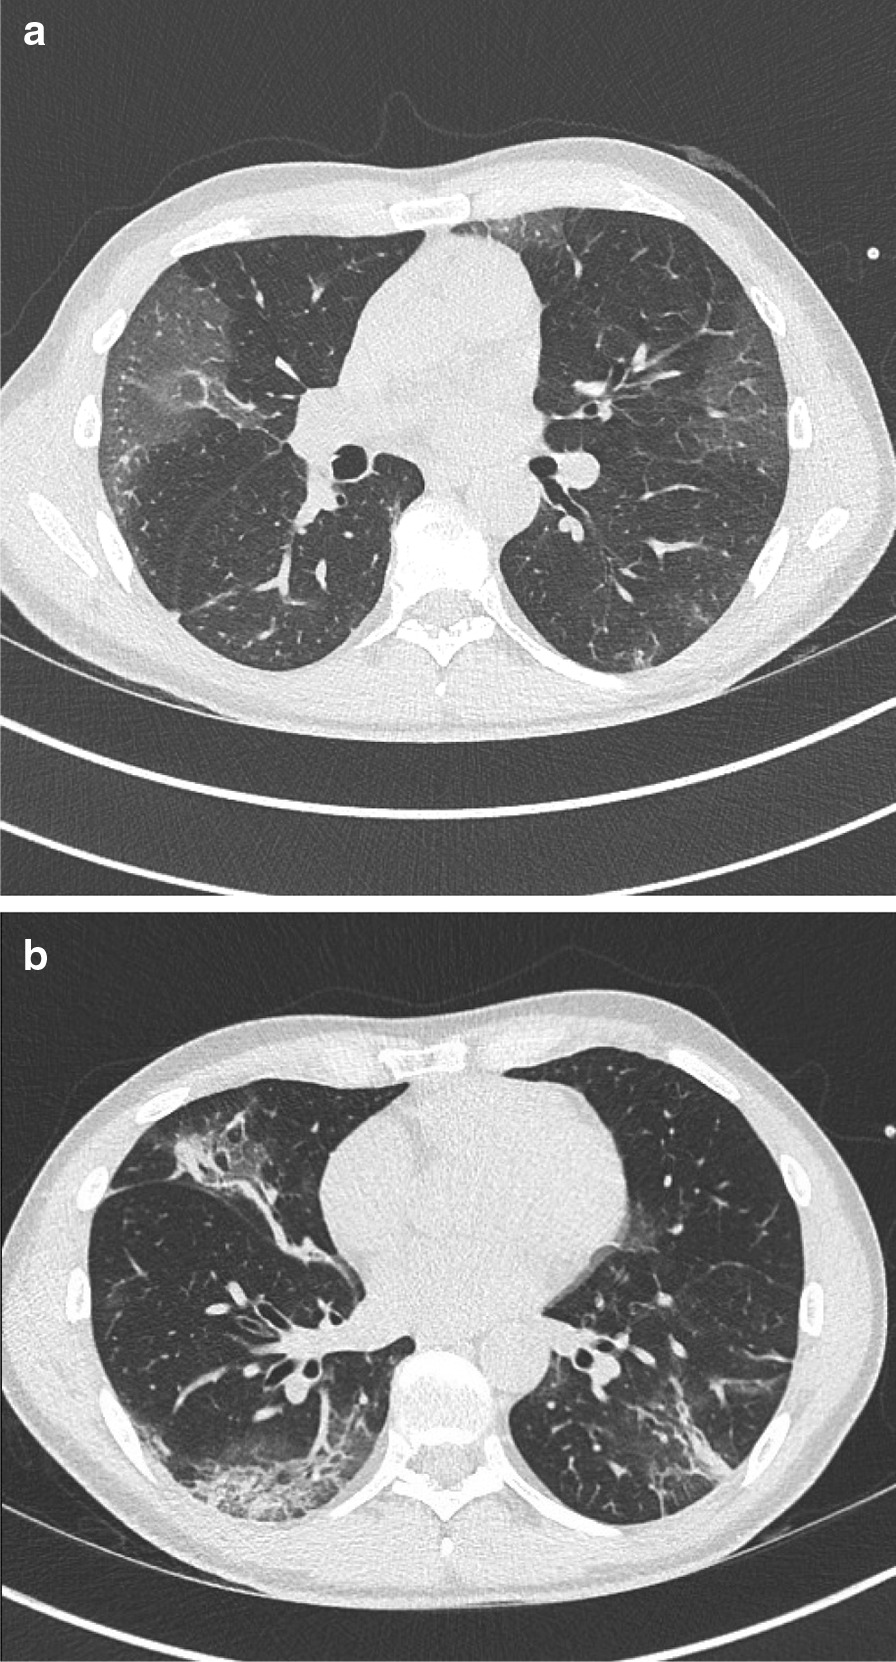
CT scan on admission. a Bilateral ground glass opacities predominantly with a peripheral lung distribution. b Crazy paving pattern in the right middle lobe and in the inferior lobes bilaterally
